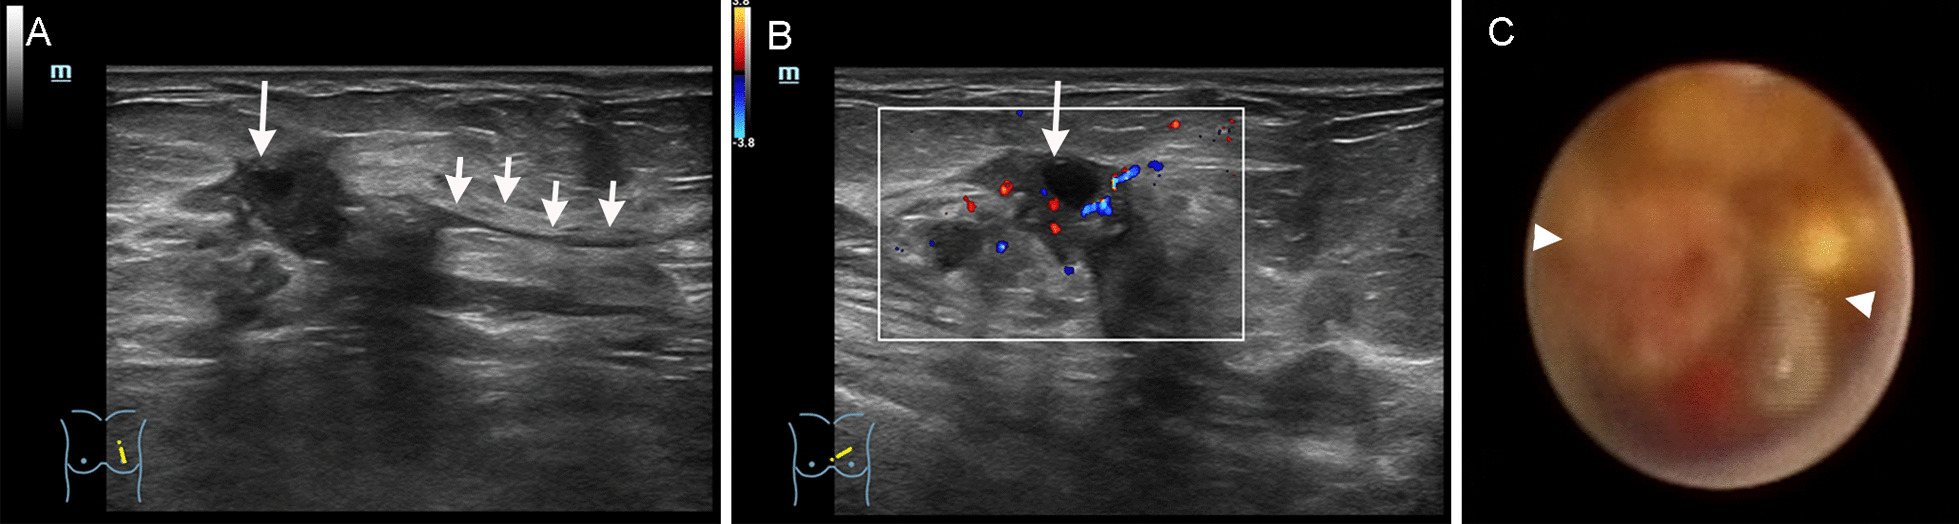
A 70-year-old female patient with bloody nipple discharge for 3 months. Comparison of ultrasound sonogram and fiberoptic ductoscopy of invasive ductal carcinoma of the breast was performed. Conventional two-dimensional ultrasound A showing a 2.2 cm × 1.2 cm cystic solid nodule (long arrow) in the gland layer approximately about 3 cm from the nipple in the 10 o'clock direction in the left breast. The nodule morphology was irregular, exhibiting “crab feet”-like margins. Adjacent duct dilation (short arrow) was observed, which extended to the nipple, showing poor intraductal sound penetration; CDFI (B) revealing punctate and short bar-like blood flow signals in the space-occupying lesion. FDS (C) revealing an irregular intraductal neoplasm completely obstructing the lumen, which bled easily when touched. Adjacent ductal walls were stiff with poor elasticity. The rear lumen was inaccessible
